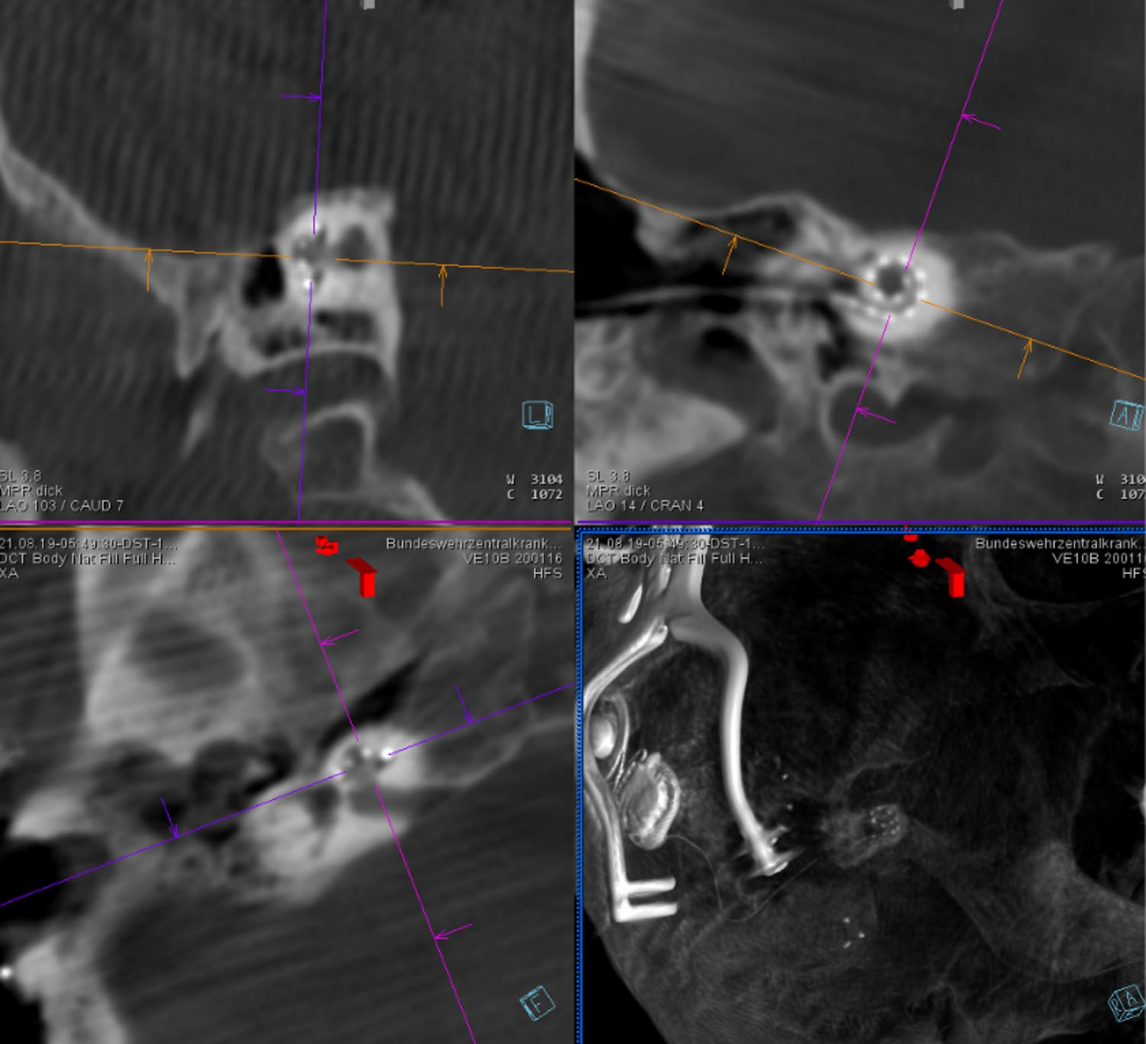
3D CT scan showing electrode position after insertion and before closure of the site
